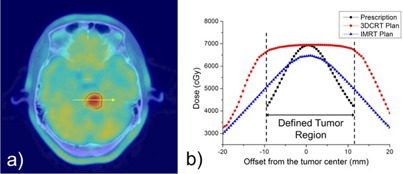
An example showing the overlay of a PET‐CT image and the tumor contour defined by the 60% of the maximum SUV (a), and the overlay profiles of the prescription and planned dose distributions from 3D CRT and IMRT plans (b).
